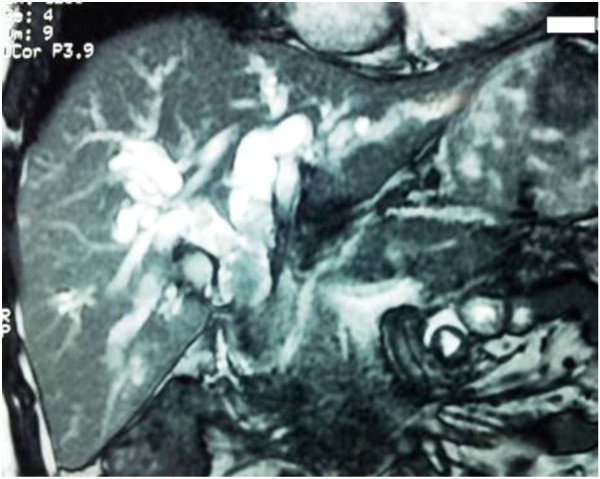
Magnetic resonance image showing a complete stop at the biliary bifurcation compatible with a Klatskin tumor associated to common bile duct stones.
